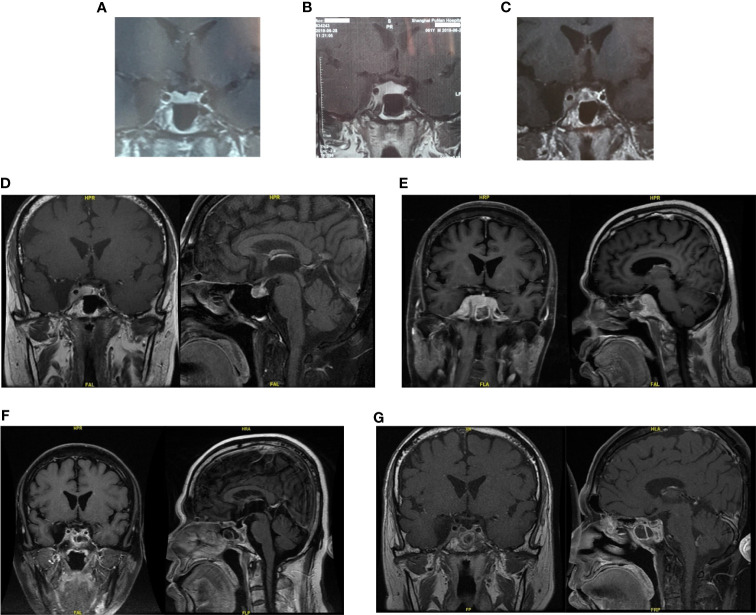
The pituitary images in different period of Case 1. (A, B) Pituitary magnetic resonance imaging (MRI) 10 and 5 months ago both demonstrated suspected “pituitary microadenoma” on the right side. (C) Three months ago, enhanced MRI showed the mass in sella turcica enlarged to 1.3 × 0.9 cm, involving the right cavernous sinus and internal carotid artery. (D) One months ago, the pituitary MRI showed the mass might be “pituitary macroadenoma” (12.7 × 6.7 × 11.1 mm, demonstrating equal T1 and equal T2 signal with slightly homogeneous enhancement) in the sellar region with the right cavernous sinus invasion (Knosp IV). (E) Pituitary MRI reexamination half months after biopsy surgery showed the mass enlarged compared with before, involving bilateral cavernous sinus. (F, G) Enhanced head MRI + DWI and Pituitary gland plain scan + enhanced MRI showed the mass was significantly smaller than before after three months’ chemotherapy.
